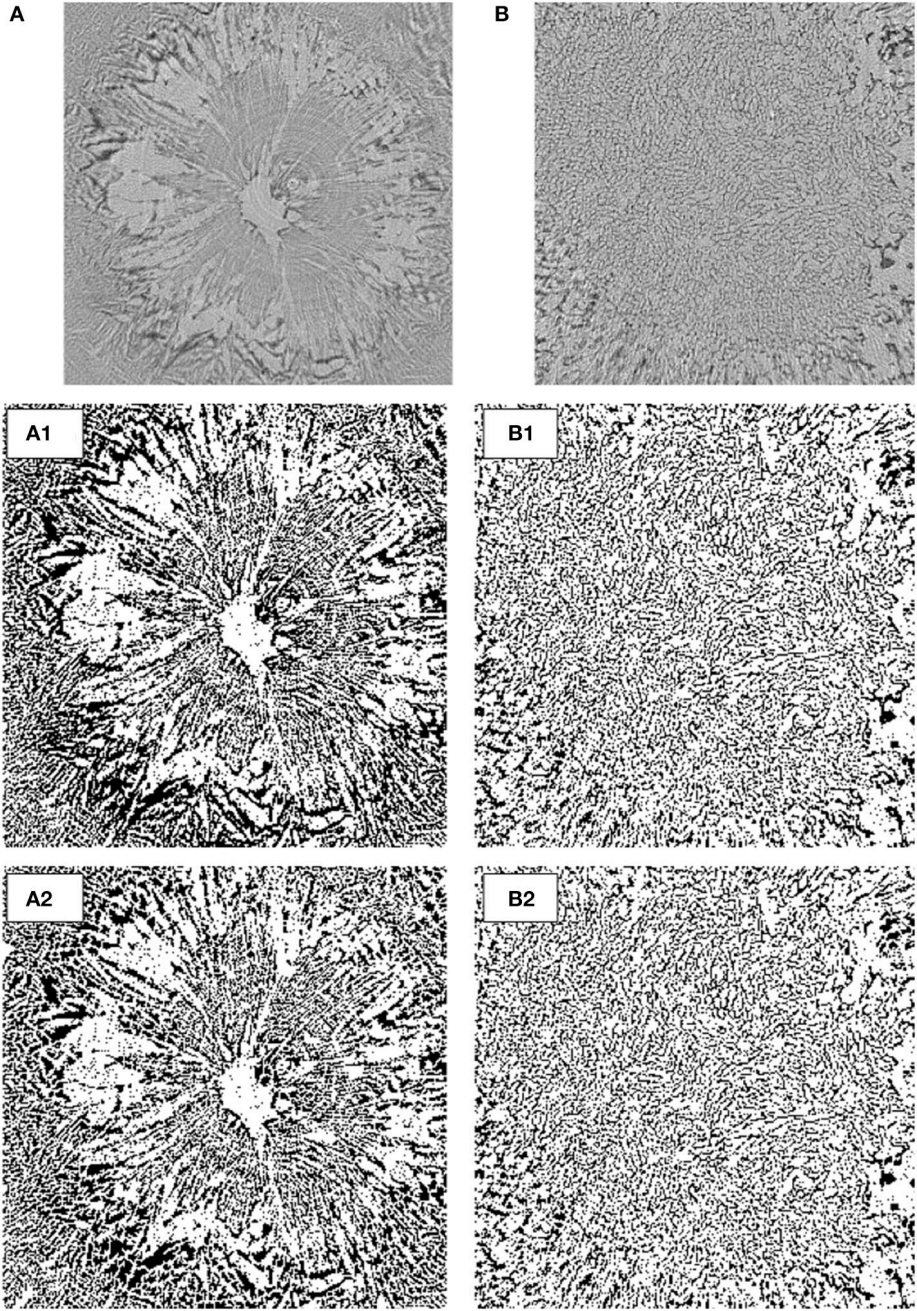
Binary images converted from cross-sectional CT images: (A) extracted from Figure 5D, (B) extracted from Figure 7D, (1) binary image, and (2) segmented by the watershed algorithm.
